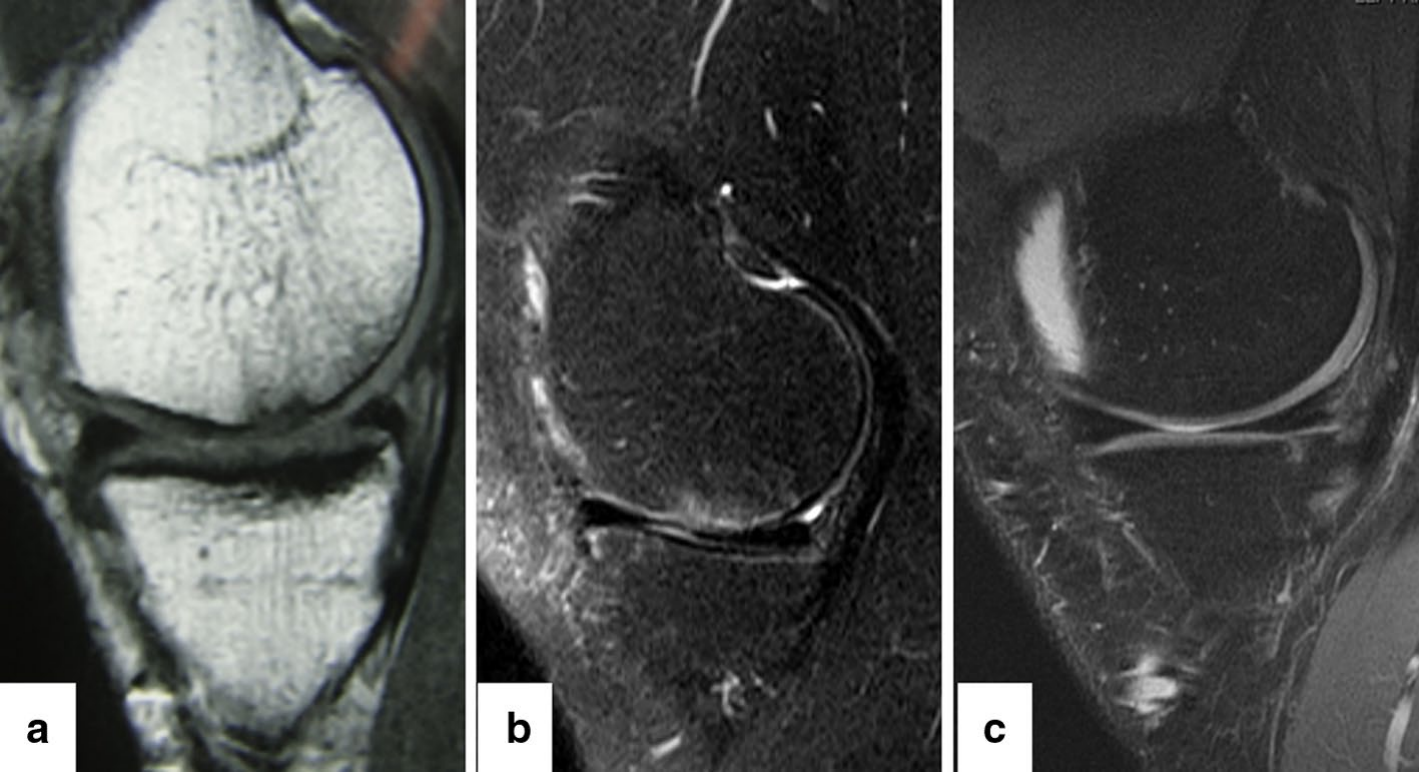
a Sagittal section magnetic resonance imaging of a grade 4 chondral lesion involving articular surface of medial femoral condyle in 50-year-old male. b 1-year follow-up MRI showing complete filling of the defect. c 5-year follow-up MRI showing establishment of smooth articular surface
